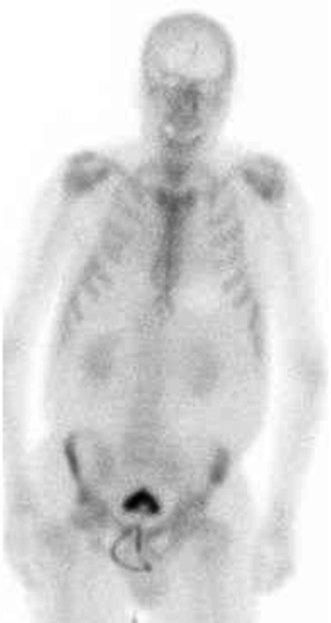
FIGURE 2 Tc‐99m scintigraphy demonstrates axial osteoblastic lesions.
